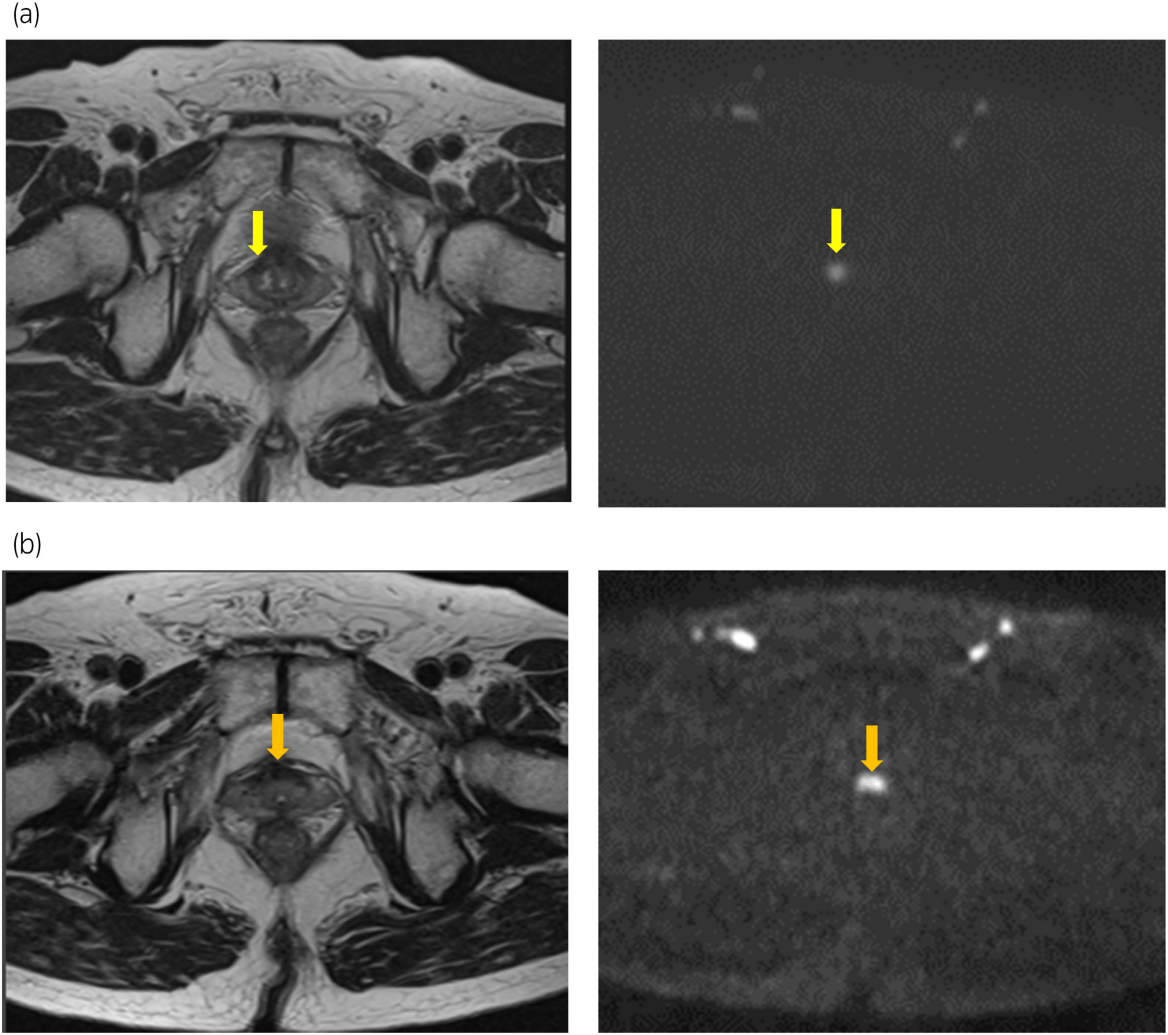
MRI images of LR. (a) MRI before salvage fLDR brachytherapy: LR (yellow arrow) was suspected on MRI because of the presence of a low signal intensity on T2‐weighted images and a high signal intensity on diffusion‐weighted imaging. (b) MRI before re‐salvage fLDR brachytherapy: LR (orange arrow), distant from the site treated with fLDR brachytherapy, was suspected on MRI.
